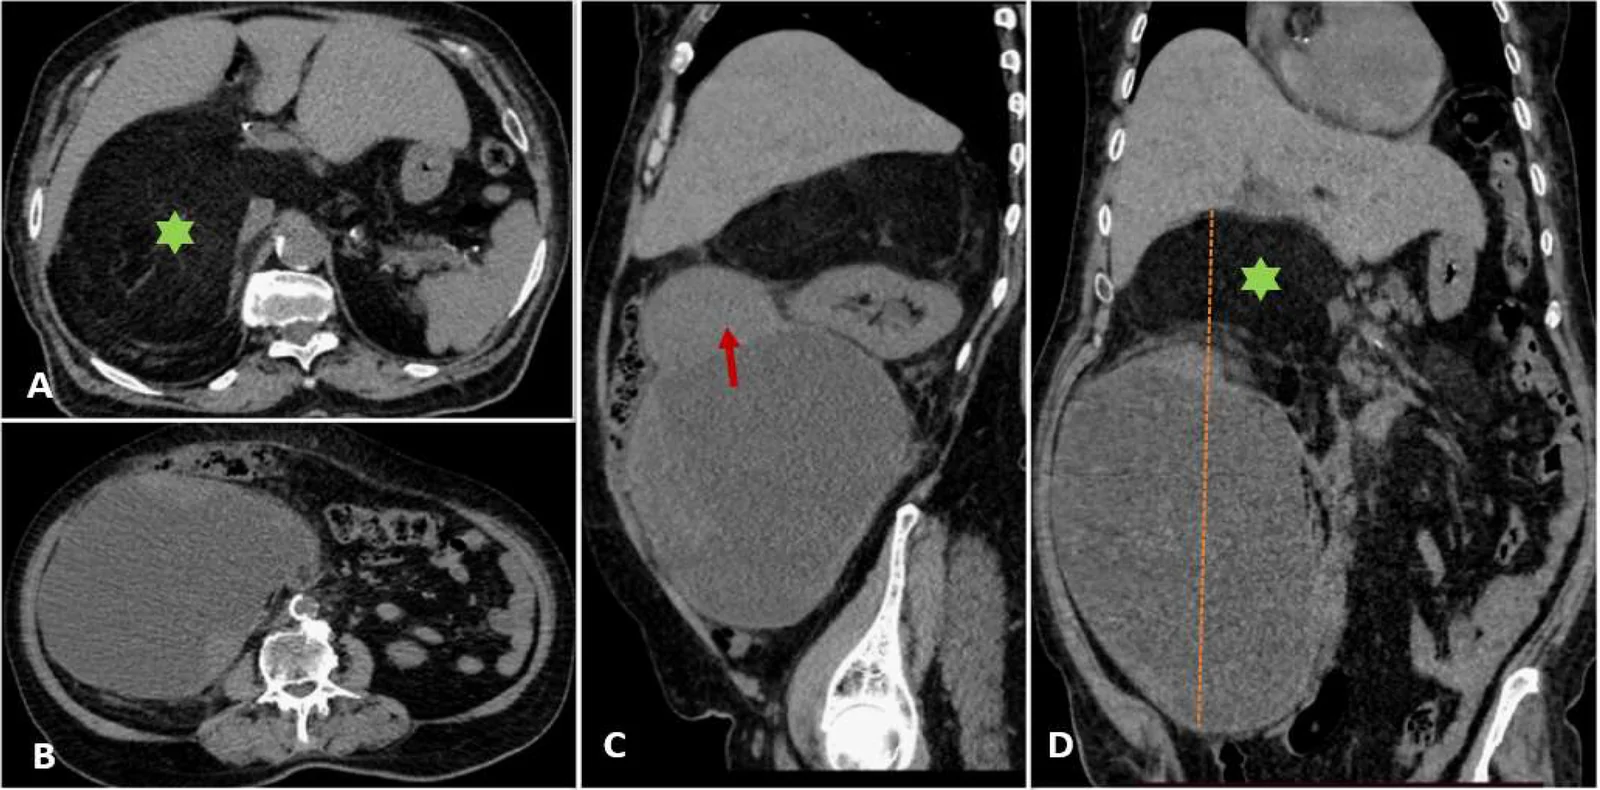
Abdominopelvic CT scan axial view (A and B) with sagital (C) and coronal (D) reconstruction, showing large retroperitoneal extensive mass with a large lipomatous portion (green star), soft tissue component (red arrow), and cystic portion.
